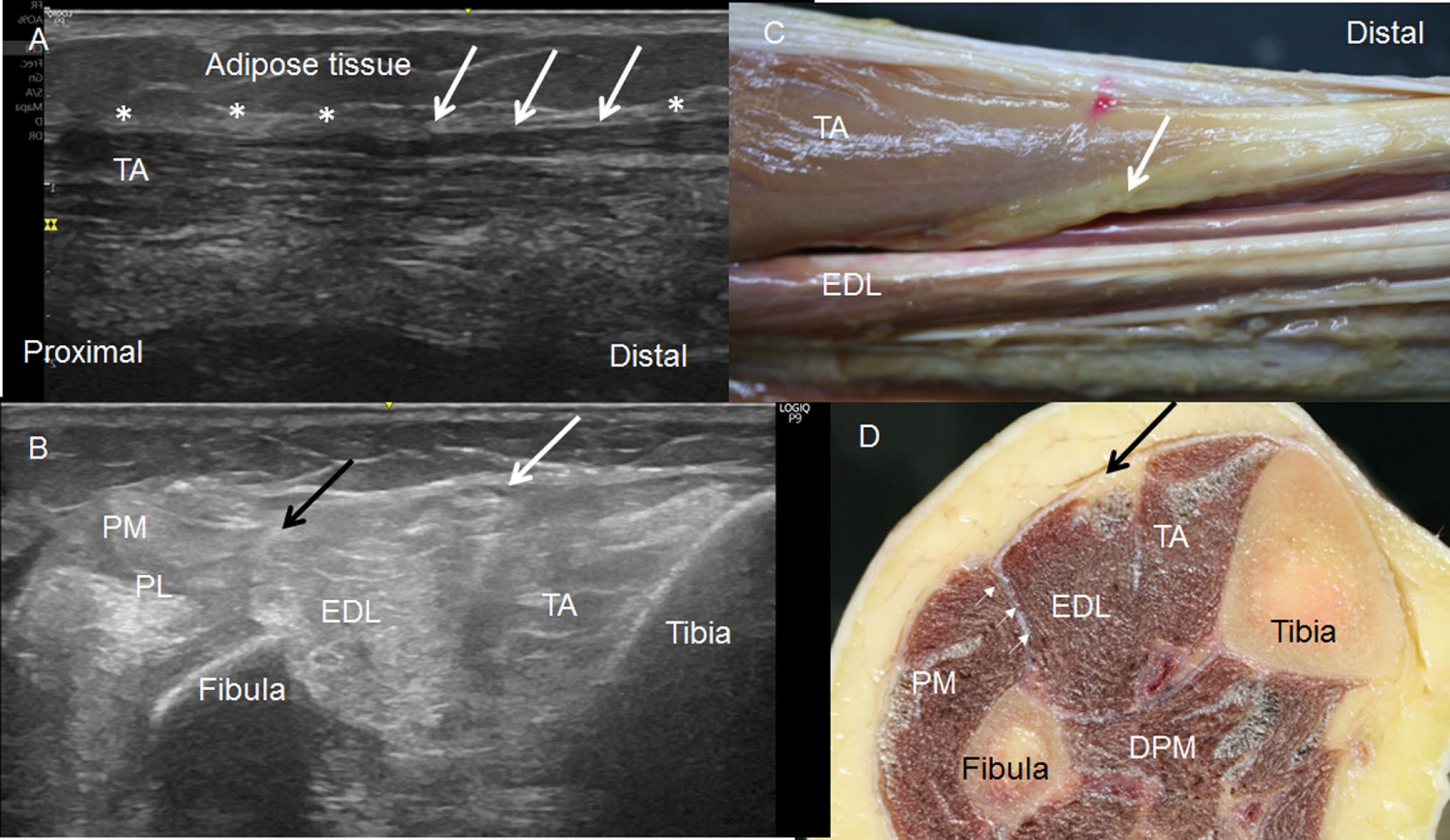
A Long-axis ultrasound view of the hypoechogenic image (white arrows) This area marks the end of the tibialis anterior muscle (TA) at the crural fascia (white asterisks). B: Short-axis ultrasound view of the leg at the middle third shows this hypoechogenicity image (white arrow) between TA and EDL. Laterally, the peroneus longus muscle (PL) is separated by a septum (black arrow) from the EDL. C: Anatomical study shows that the hypoechogenicity image corresponds to adipose tissue (white arrow) between TA and EDLof a right leg. D Transversal cut of the leg, at the middle third, shows the adipose tissue (black arrow) between the TA and EDL. Laterally, the peroneus muscles (PL) are separated from the EDL by a septum (short white arrows). Posteriorly the deep posterior muscles of the leg (DPM)
